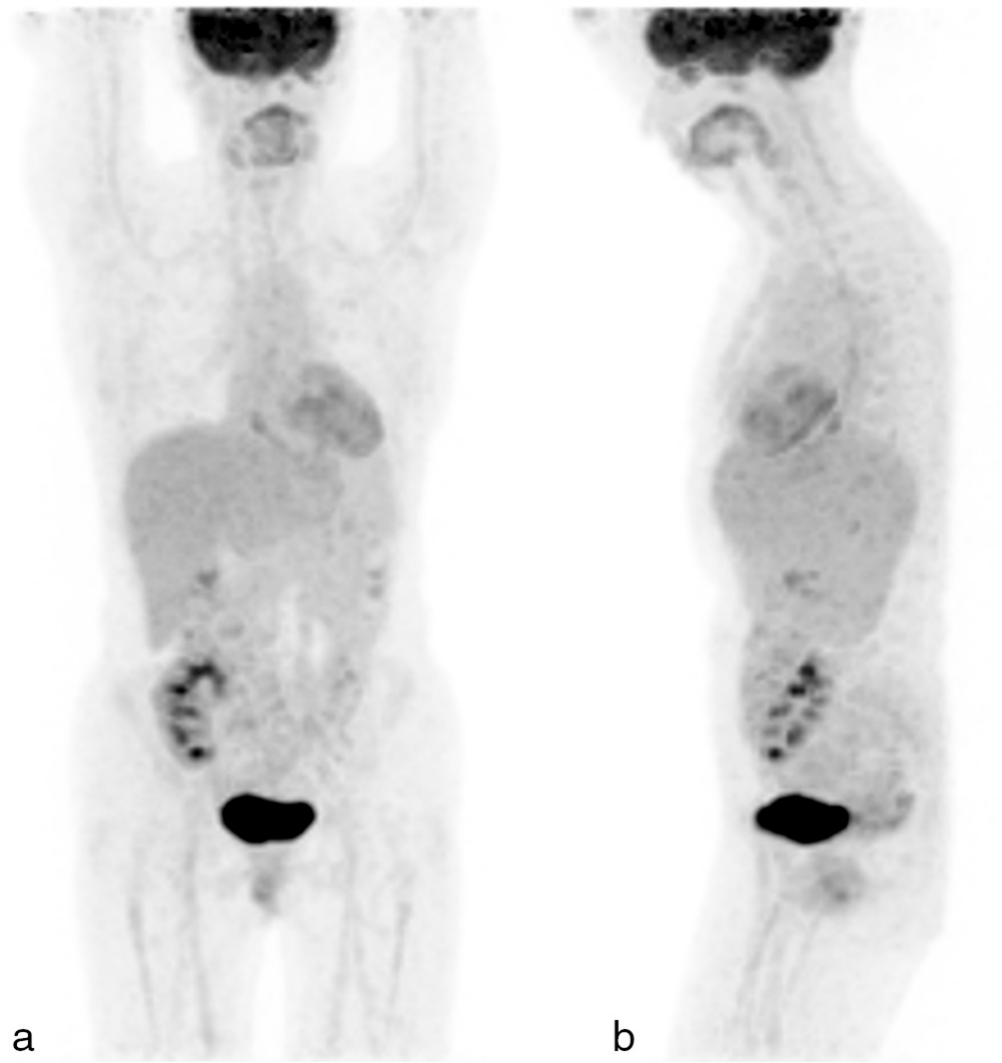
A follow-up ^18^F-fludeoxyglucose positron emission tomography/CT performed 4 weeks after completion of the fourth cycle of rituximab. (a) Anterior and (b) lateral maximum intensity projection images showing complete metabolic resolution of all of the lung nodules as well as the Epstein–Barr virus-positive oesophageal ulcer.
